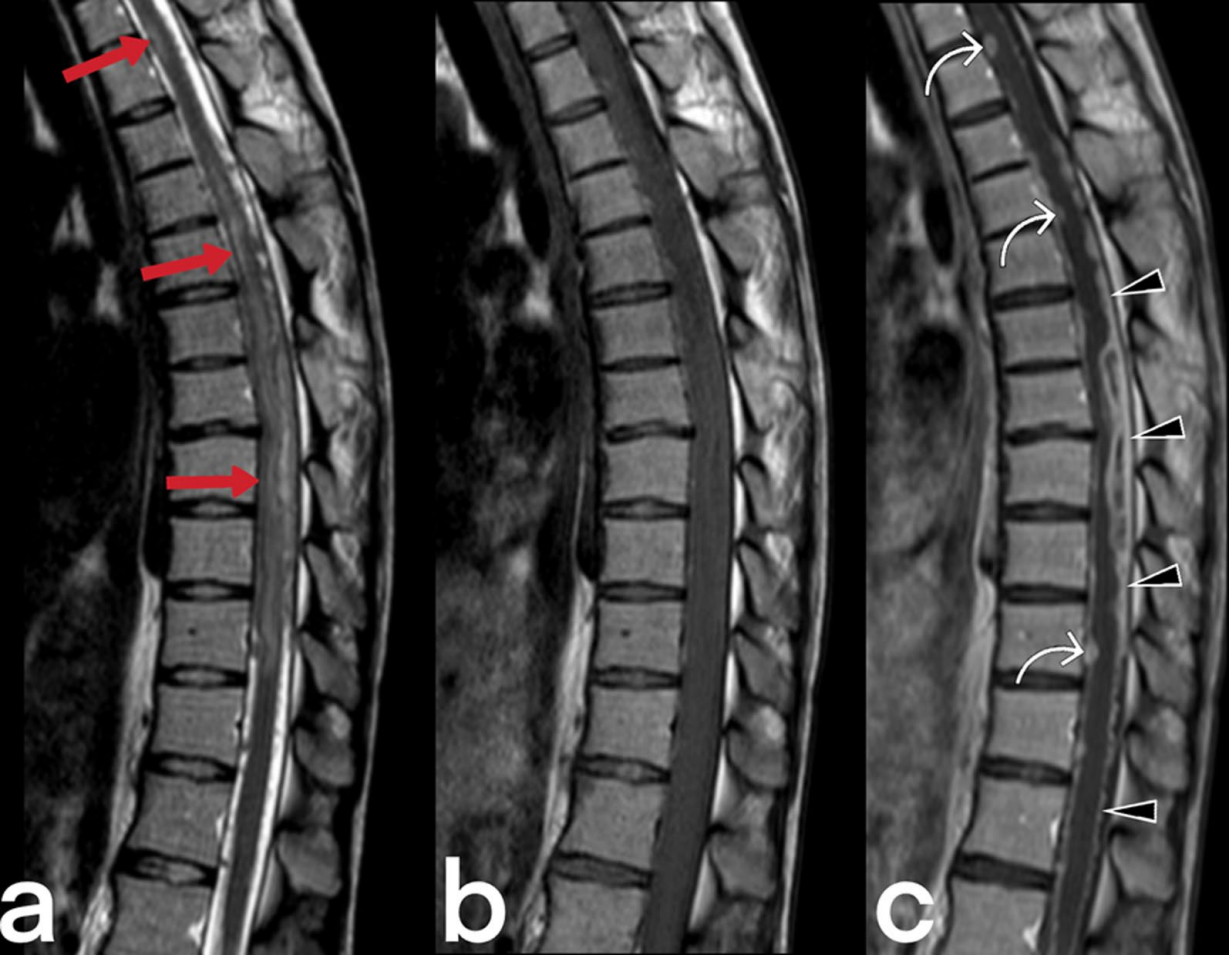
MR images of thoracic vertebrae of a 23-year-old female. Sagittal T2-weighted image reveals a heterogeneous signal increase in the spinal cord (red arrows) (a). Precontrast (b) and postcontrast (c) T1 weighted images of the same patient show linear (arrowheads) and nodular (curved arrow) dural enhancement and tuberculous abscess formations in the posterior epidural space
